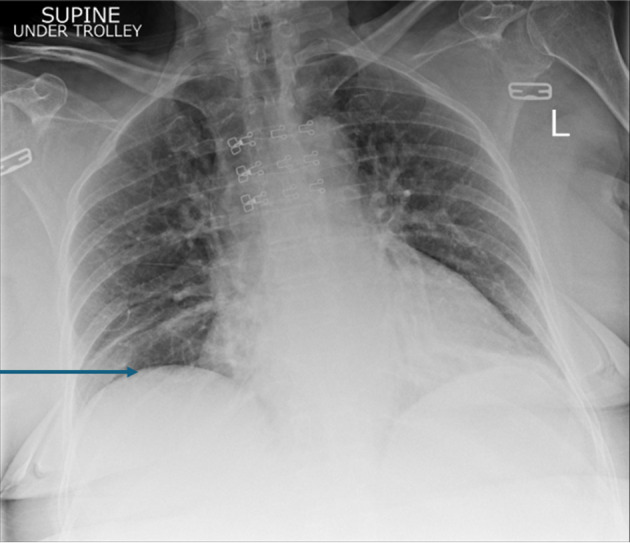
Initial supine chest radiograph (CXR) revealed shallow lung expansion with elevated hemidiaphragms more in the right side. The arrow points towards elevated right hemidiaphragm. Image taken preoperatively.
